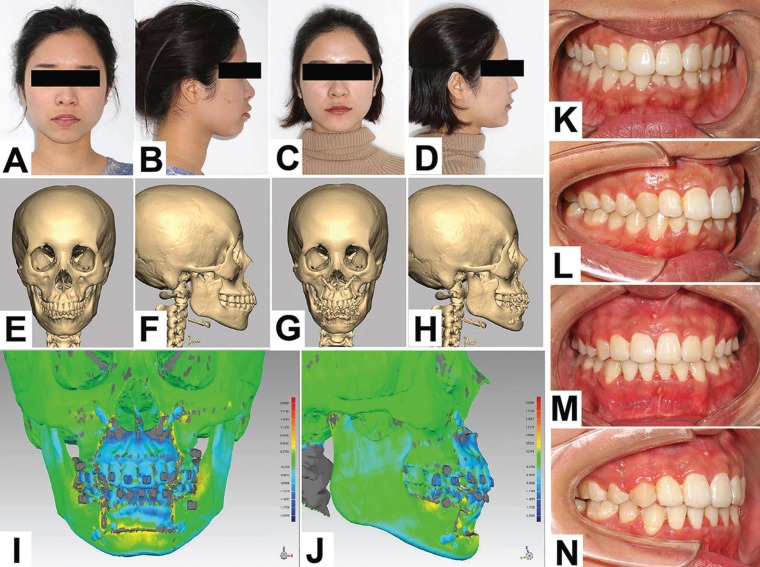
The case pictures of a representative patient who complained of an unfavorable face type for ten years. A-D: The facial profiles before (A, B) and after (C, D) the template guided ASO; E-H: The 3D reconstructed spiral CT images before (E, F) and after (G, H) the template guided ASO; I and J: The superimposed 3D images of virtual and actual outcomes of the representative patient; K-N: The contrast of preoperative (K, L) and postoperative (M, N) occlusion of the representative patient.
